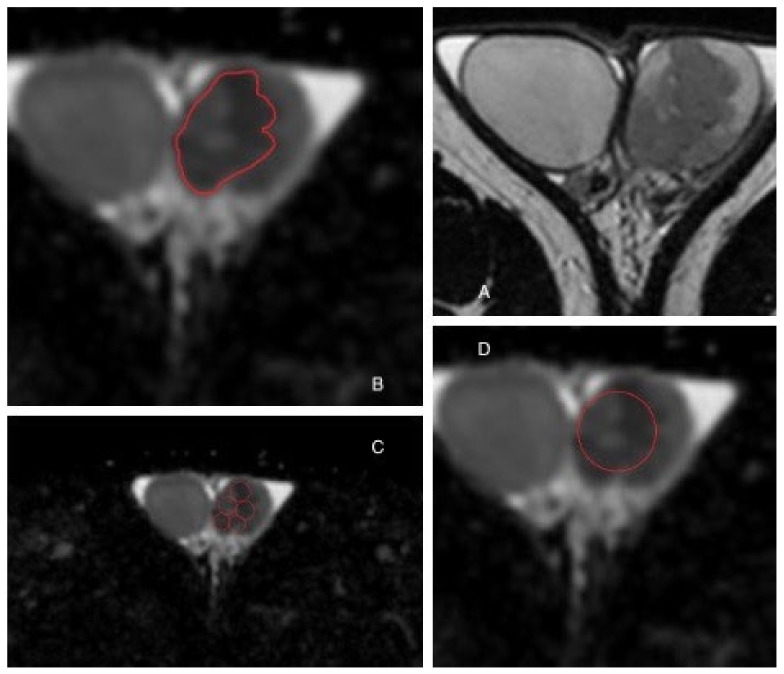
Testicular tumor in a 36-year old male. (A) is an MRI of both testicles. (B) is *whole volume* ROI. (C) is *small* ROIs. (D) is *round* ROI.
